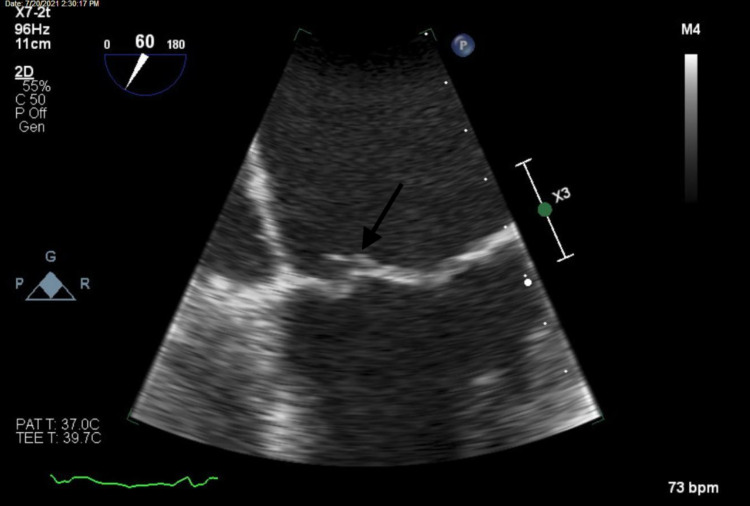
Transesophageal echocardiogram, midesophageal four-chamber view, revealing a small, mobile density attached to the anterior mitral valve leaflet.
